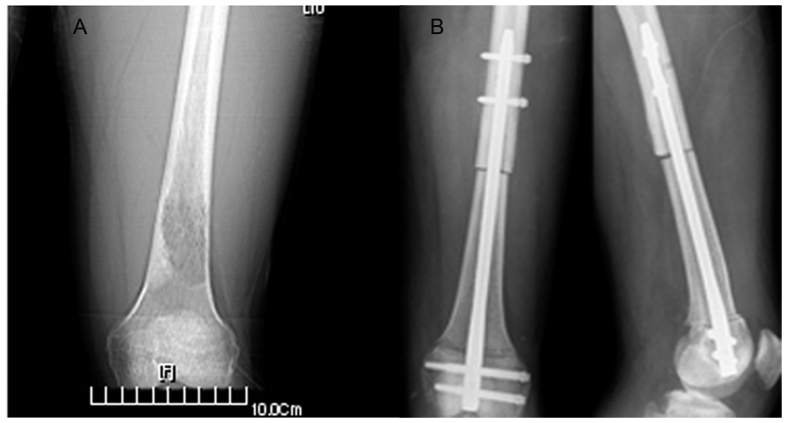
X-ray image showing that the interlocking intramedullary nails were excellent, with high stability. (A) Preoperative and (B) postoperative radiographs (Left, front view; right, lateral view).
